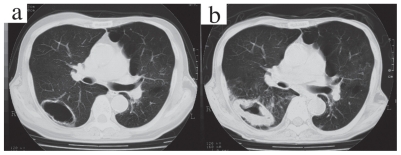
Chest CT in 2003 (a) demonstrated a CT thick-walled cystic lesion in the superior segment of the lower lobe of the right lung. A corresponding CT image in 2004 (b) demonstrated increased thickening of the wall.
